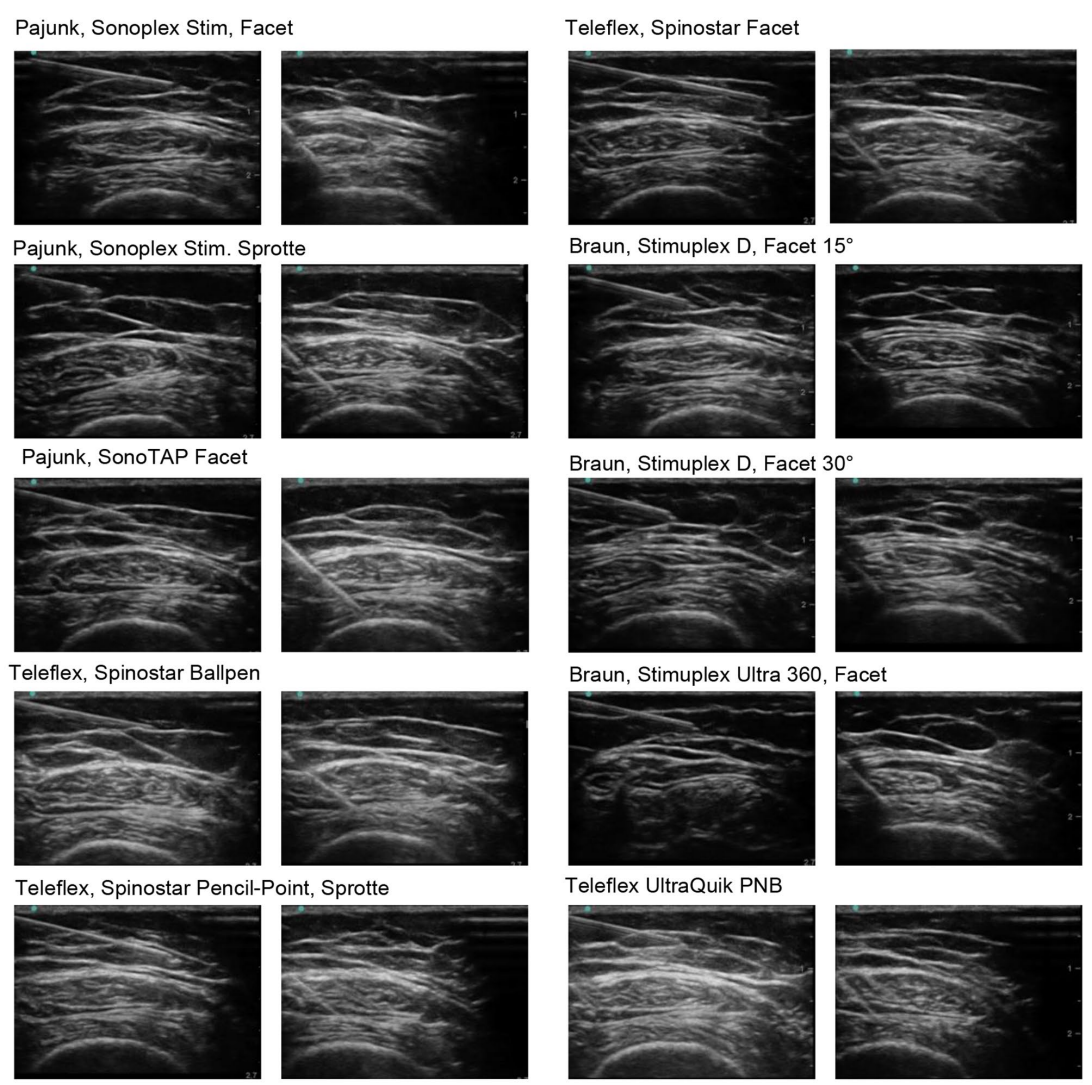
Examples of ultrasound images for all needles for insertion angle 10° (first and third column) and 45° (second and fourth column)
